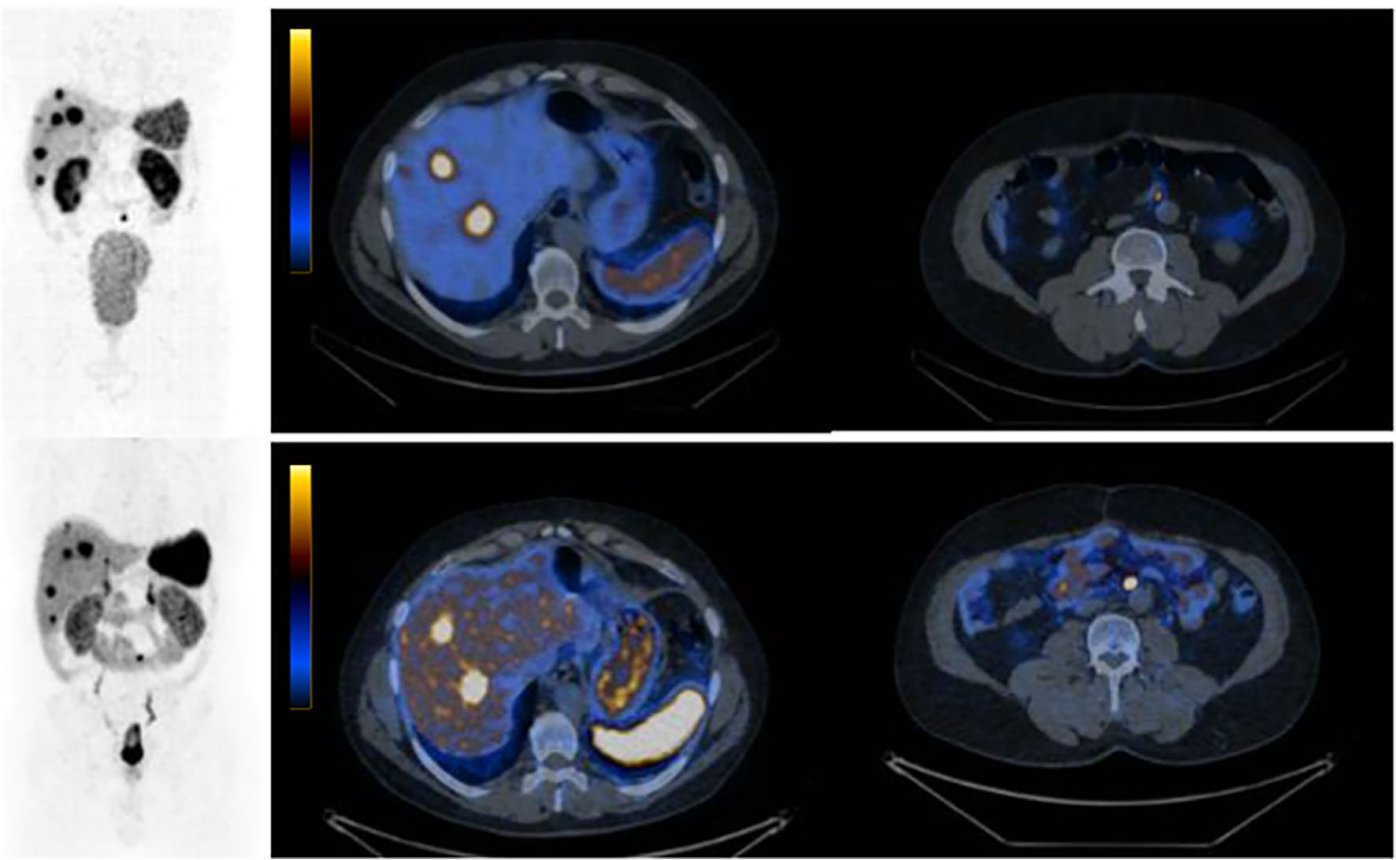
Fig. 1 Maximum intensity projection (MIP) and axial fused images: upper line -[^99m^Tc]Tc-TECANT1 SPECT/CT (4 h post injection, injected activity 785 MBq, 120 frames, 20 s per frame); bottom line — [^68^ Ga]Ga-DOTA-TATE PET/CT (1 h post injection, injected activity 146 MBq; 3 min per bed). Scans were obtained within 13 days. Long-acting somatostatin analogue was withdrawn 4 weeks before imaging
